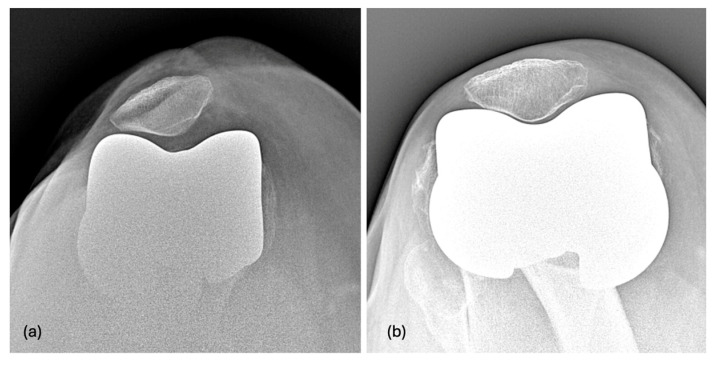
Difference between the patellar tilt of a mechanical alignment-designed femoral component (PTA = 6°) and a kinematic alignment-designed femoral component (KA_D_FC) (PTA = 20.6°): (a) Postoperative 30° axial radiograph of an unrestricted KA mechanical alignment-designed femoral component with a prosthetic trochlear angle of 6°. The prosthetic trochlear angle is medial to the QLF. As observed in the radiograph, the patella appears to be in a position of external patellar hyperpressure. (b) Postoperative 30° axial radiographs of an unrestricted KA-designed femoral component with a prosthetic trochlea angle of 20.6°. The prosthetic trochlear angle is lateral to the QLF. As observed in the radiograph, the patella is centered in the trochlear groove.
